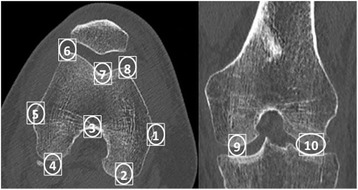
Location of landmarks on axial (left) and frontal (right) CT scan slices: 1) medial epicondyle, 2) most dorsal point on medial condyle, 3) top of intercondylar notch, 4) most dorsal point on lateral condyle, 5) lateral epicondyle, 6) most ventral point on lateral edge of trochlear groove, 7) most distal point at bottom of the trochlear groove, 8) most ventral point on the medial edge of the trochlear groove, 9) most distal point on medial condyle, 10) most distal point on lateral condyle
